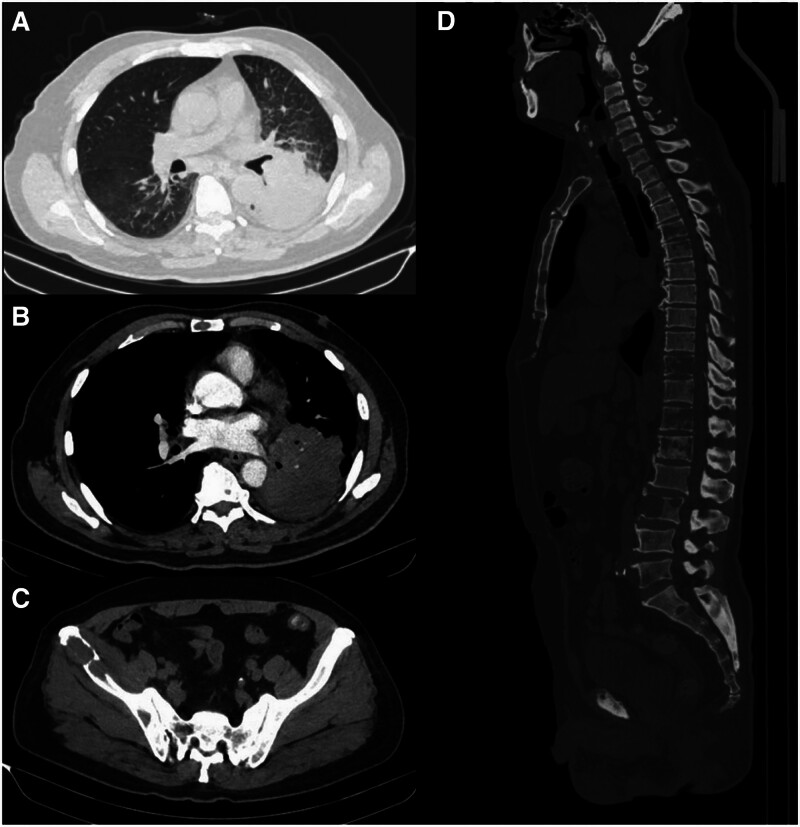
A 16-slice computed tomography (CT) scan revealed a space-occupying lesion with obstructive pneumonia in the lower lobe of the left lung (9.8 × 6.9 cm) and osteolytic destruction. (A) The space-occupying lesion in the lung window; (B) the space-occupying lesion in the mediation window; (C) osteolytic destruction of the right ilium; (D) osteolytic destruction of the vertebral body.
